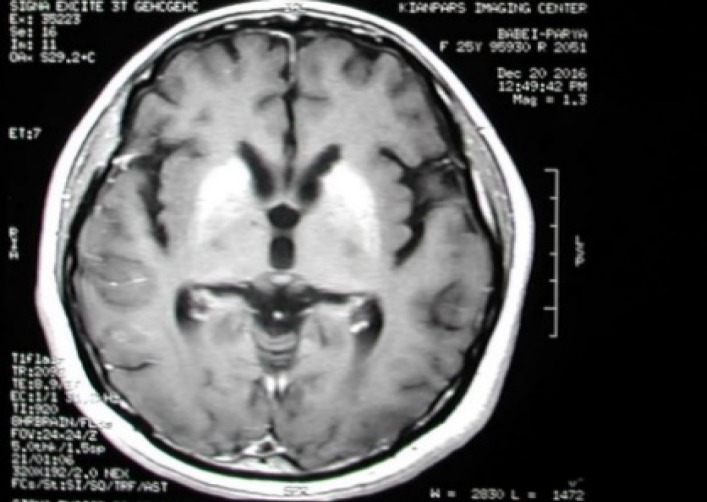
FLARE image in magnetic resonance imaging (MRI) of brain at the beginning of multiple sclerosis (MS) diagnosis
